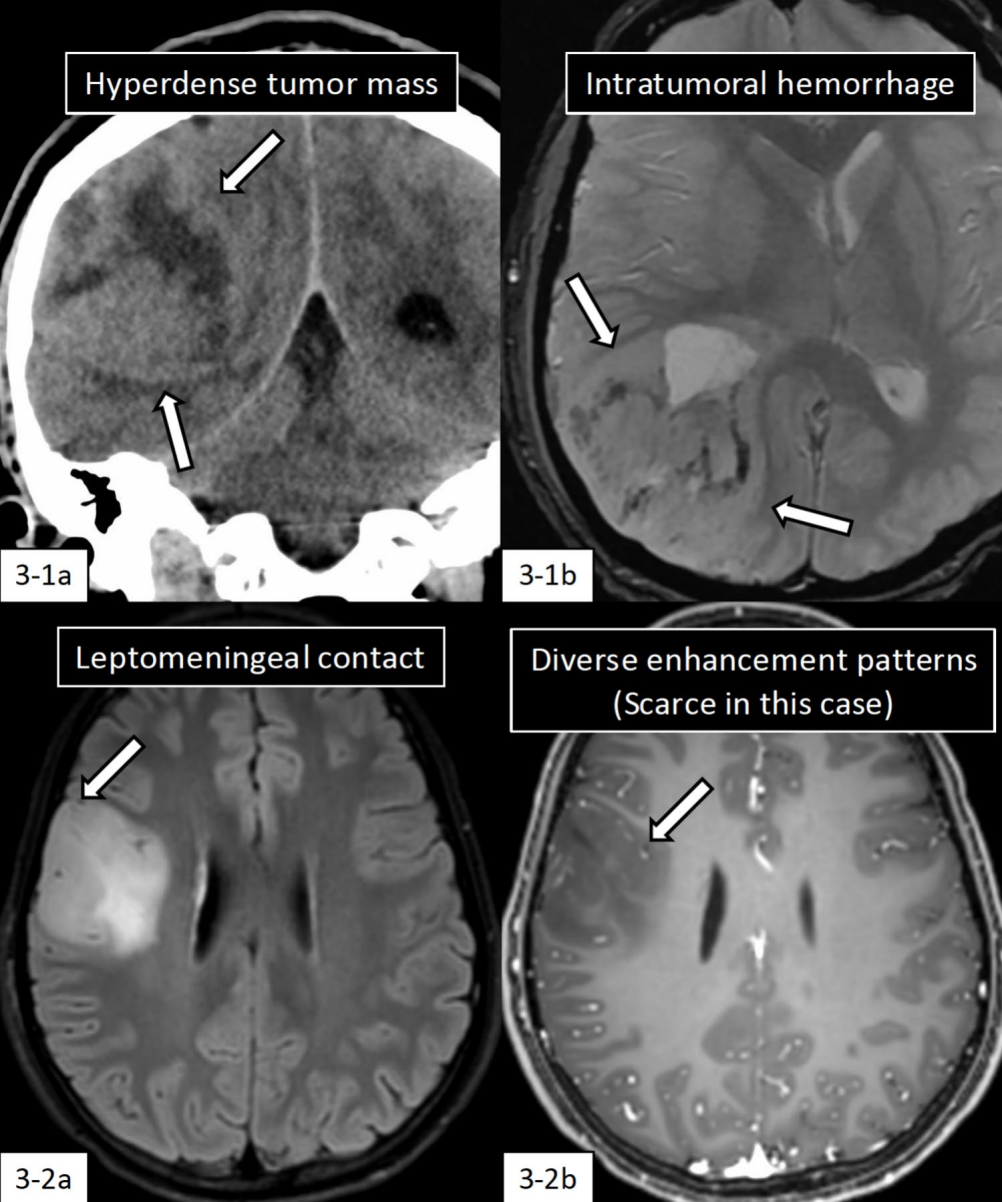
Imaging features of diffuse hemispheric glioma, H3 G34-mutant. 3-1 (22 years old, man): A large lobulated tumor mass involving the cortex with leptomeningeal contact shows hyperdensity with central necrosis on non-enhanced computed tomography coronal image (3-1a, arrows). Intratumoral hemorrhage is observed on T2*-weighted imaging (3-1b, arrows). 3-2 (16 years old, girl): A circumscribed tumor mass involving the cortex with leptomeningeal contact (3-2a, arrow) in the right frontal lobe shows hyperintensity on fluid attenuated inversion recovery image with a scarce contrast enhancement (3-2b). The same cases with different images have been evaluated in our previous study [53]
